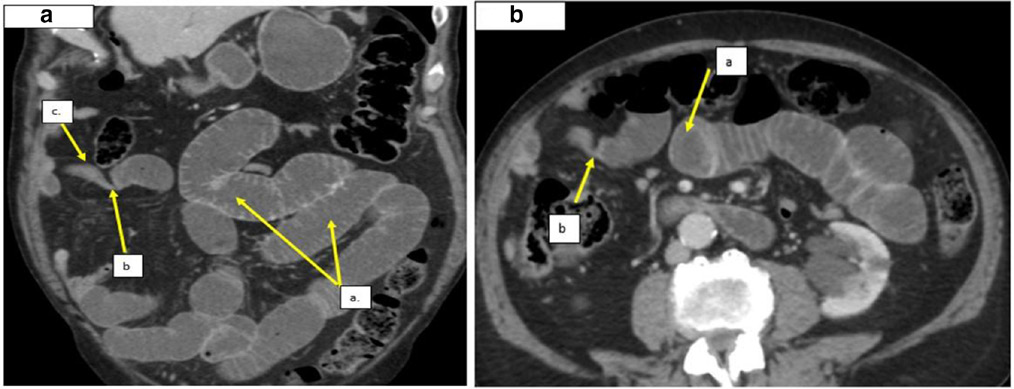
a. Axial CT images and Figure 4b, Coronal CT images, demonstrating: (a) dilated fluid filled small bowel loops upstream to the acute transition point. a. Fat density band indenting the bowel lumen at the acute transition point. b. Collapsed small bowel distal to the transition point.
